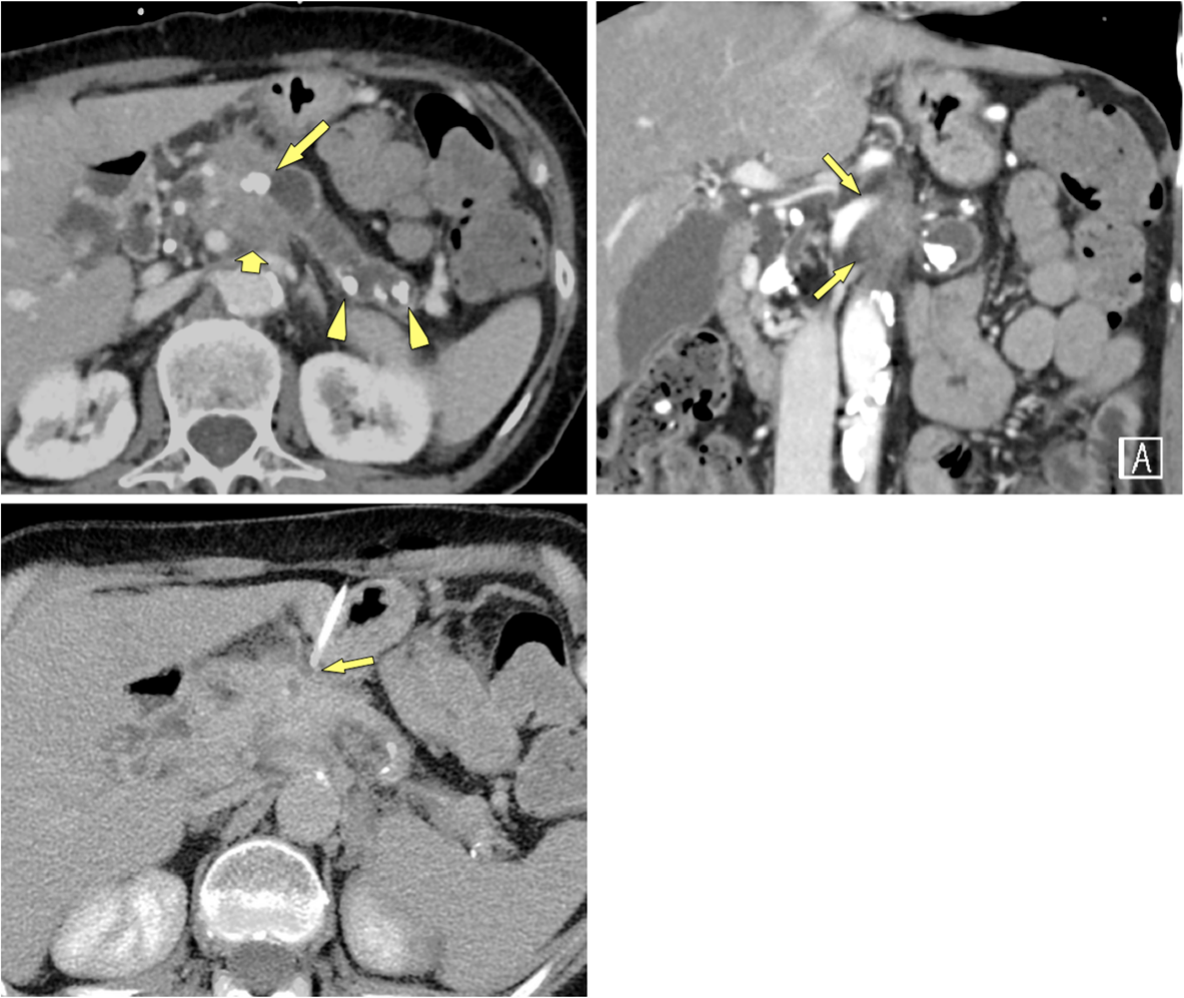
Soft tissue mass with vessel encasement in calcified CP: locally advanced PDAC (66 y, female). a Contrast-enhanced MDCT shows signs of severe CP with parenchymal calcifications (arrowheads) and a large intraductal stone (arrow) with duct dilatation. However, there is a non-calcified soft-tissue mass present, which infiltrates into the mesenteric root (thick arrow). b Coronal reformation shows encasement of the superior mesenteric artery by the mass (arrows), which is highly suspicious for PDAC. c Diagnosis was confirmed by percutaneous CT-guided transgastric biopsy (arrow)
